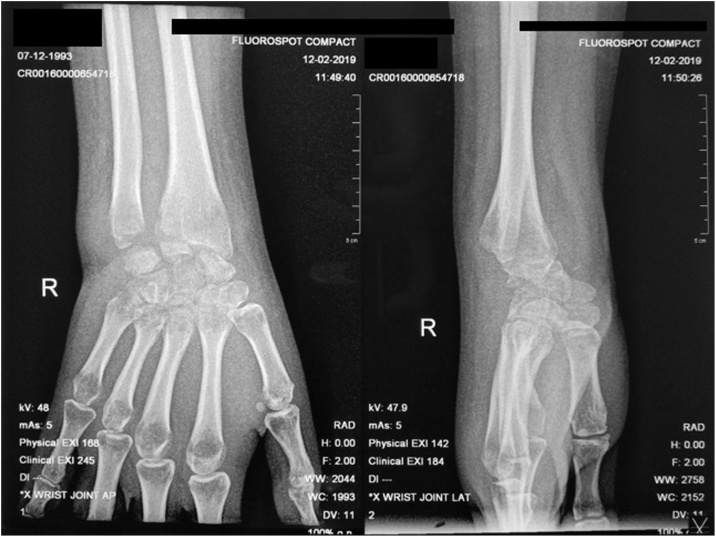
X-Ray Examination of the Wrist.
